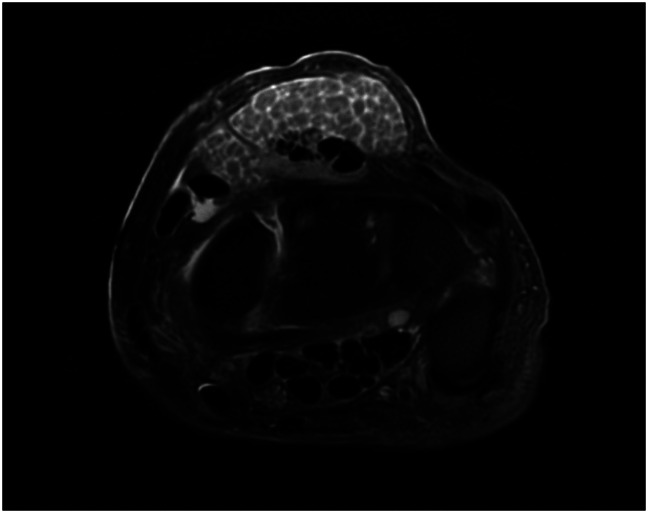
T2-weighted axial MRI of the right wrist demonstrate innumerable rice bodies within the fourth dorsal compartment with distension of the tendon sheath and a partial rupture of the extensor digitorum communis.
